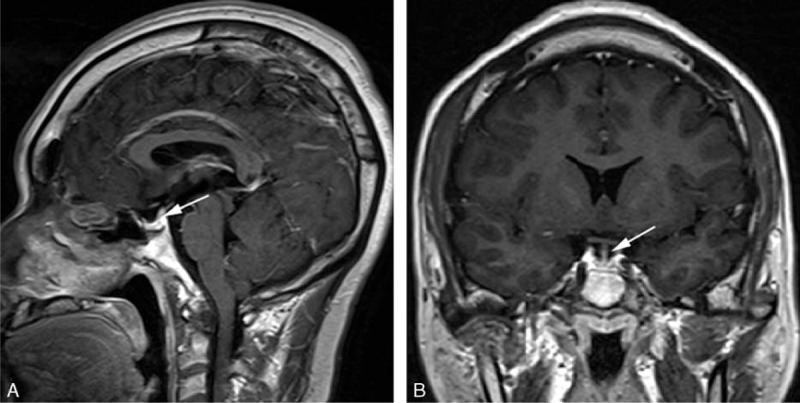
Head magnetic resonance imaging 2 years after surgery in July 2015: postcontrast coronal image shows no delayed enhancement in the saddle region (white arrow).
